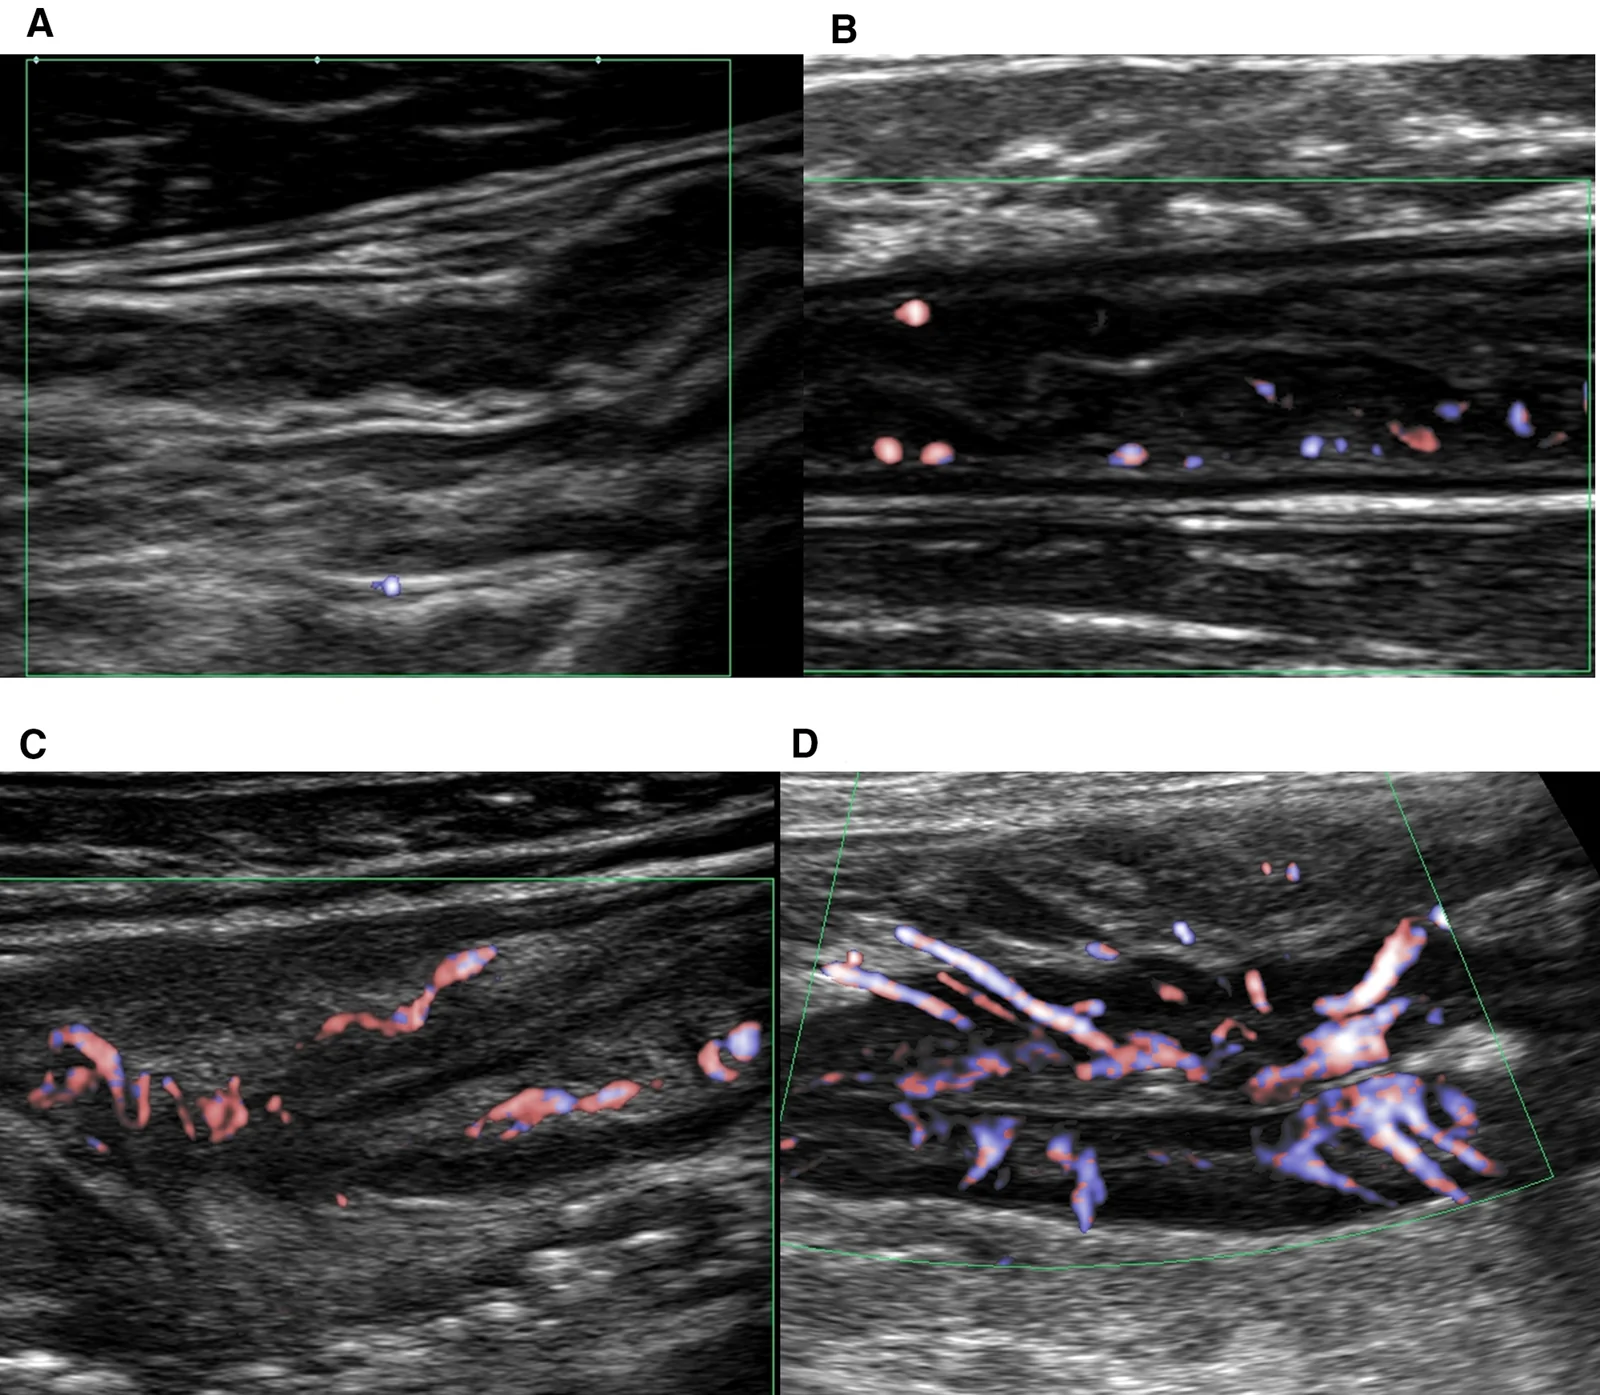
Representative examples of 4 SMI grades using intestinal ultrasonography. Grade 0: no increased vascularity (A). Grade 1: short stretches of vascularity as spots (B). Grade 2: longer stretches of vascularity (C). Grade 3: longer stretches of vascularity reaching the mesentery (D). SMI = superb microvascular imaging.
